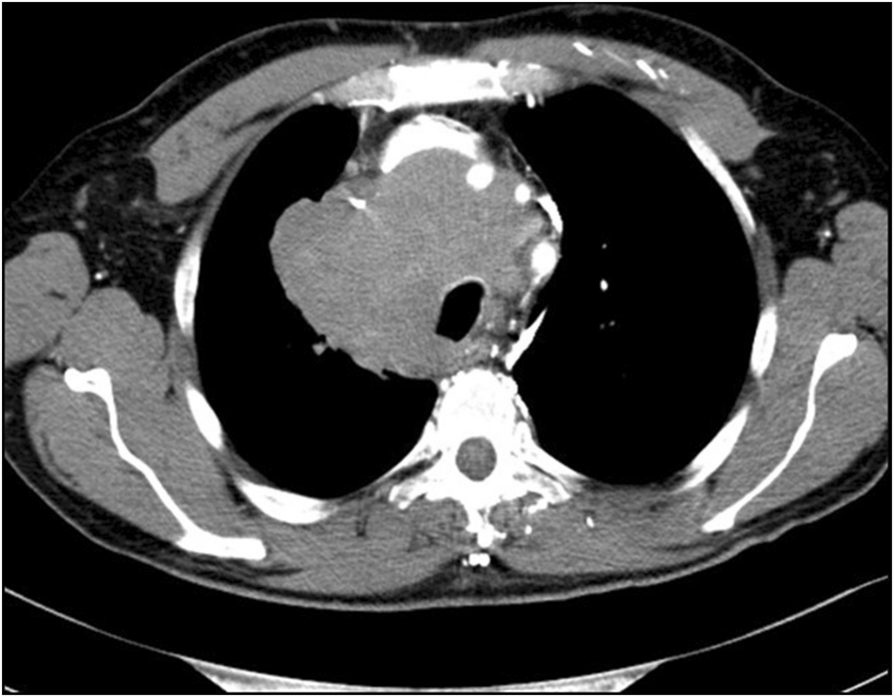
Computed tomography image of the mediastinal mass intermingled with mediastinal lymphadenopathy
